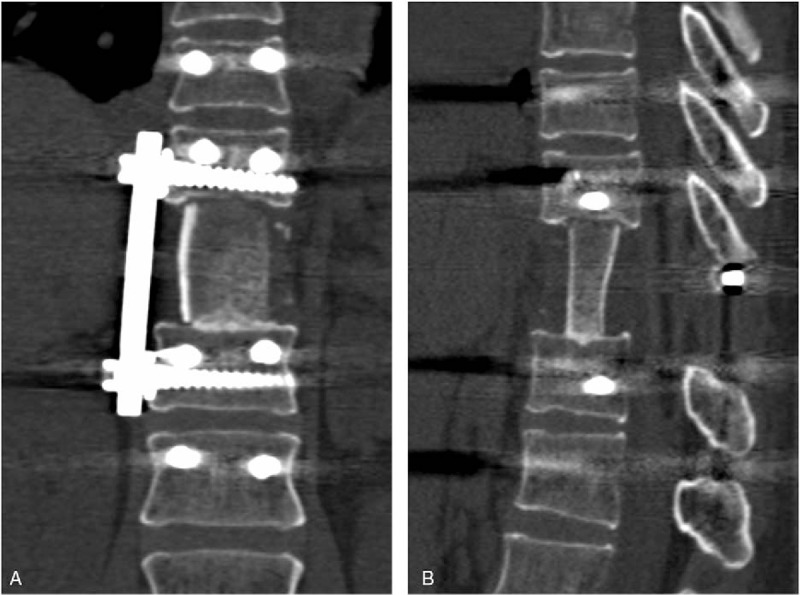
Postoperative 3-dimensional computed tomography (CT) scan images in the 6 months. (A) Coronal CT; (B) sagittal CT.
